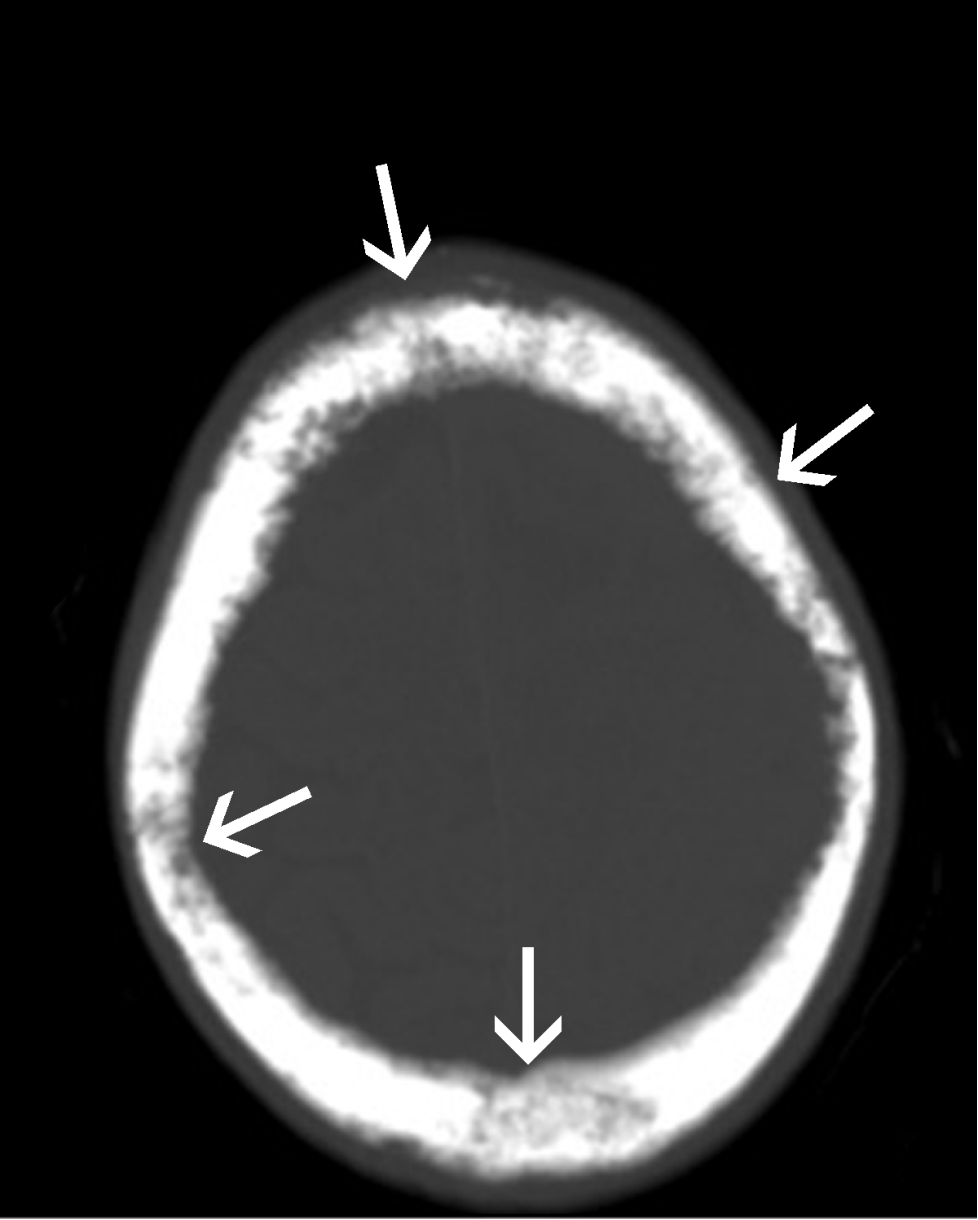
CT scan revealing diffuse lytic/blastic lesions of the skull - arrows.
